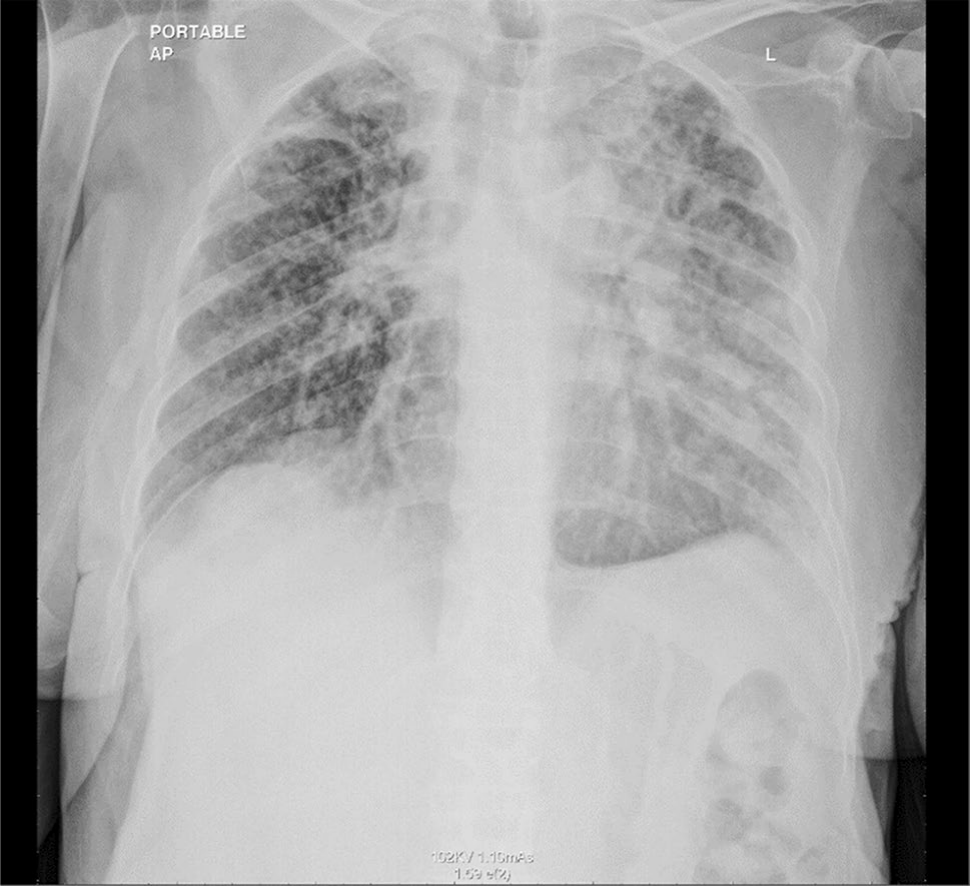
Chest X-ray showed new bilateral reticular and nodular pulmonary infiltrates.
